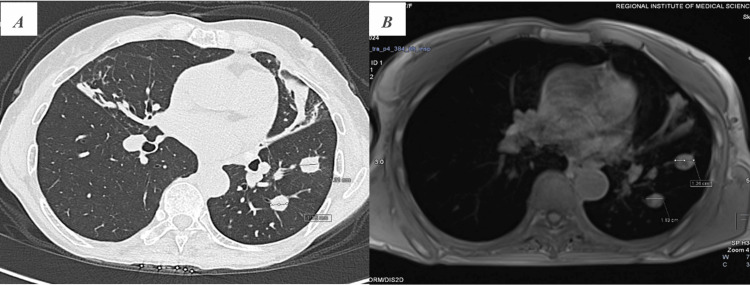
(A) Axial view of HRCT of the thorax showing well-defined pulmonary nodules in the LLL anteromedial and posterior segments. (B) T1-VIBE MRI sequence; corresponding nodules appear hyperintense in the same LLL segments, demonstrating reliable localization. Note: not all nodules visible on CT (Panel A) are identifiable on MRI (Panel B), consistent with the size-dependent detection limitation of MRI for sub-5 mm lesions. Right lung findings were separately evaluated in additional cases. HRCT = high-resolution computed tomography; LLL = left lower lobe; T1-VIBE = volumetric interpolated breath-hold examination; MRI = magnetic resonance imaging
